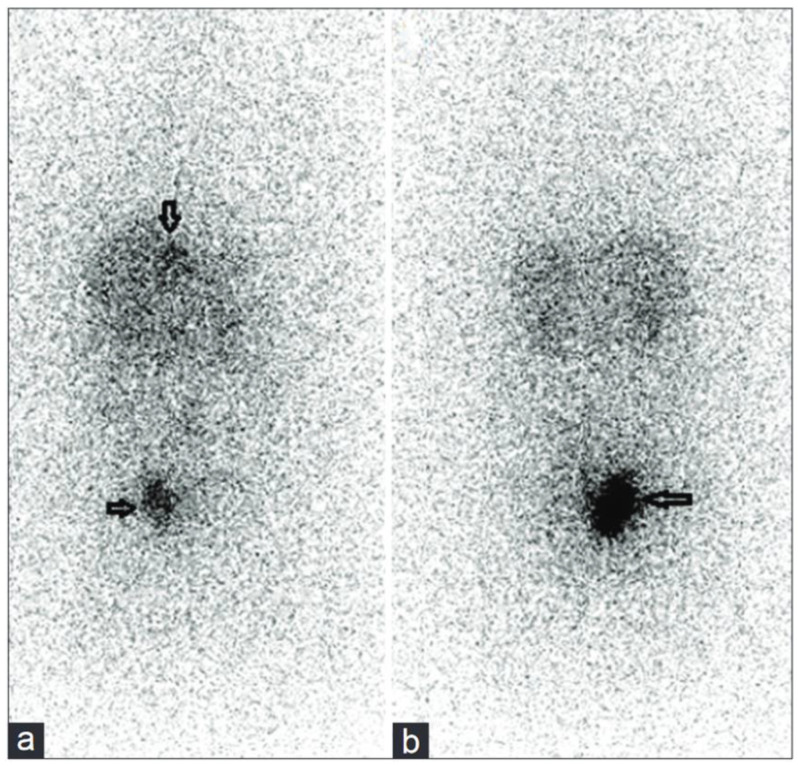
Anterior (a) and posterior (b) post-therapy whole-body SPECT/CT scans showing intense accumulation in pararectal lesion (horizontal arrows) and liver metastases (vertical arrows) in a patient with a grade 2 NET, 24 h after treatment with 5.5 MBq [^225^Ac]Ac–DOTATATE, acquired for 30 min using 256 × 1024 matrix and high-energy general-purpose collimators (218 keV and 440 keV photon energies with 20% window width), showing increased uptake in pararectal lesions, lymph nodes, and liver metastases [82].
